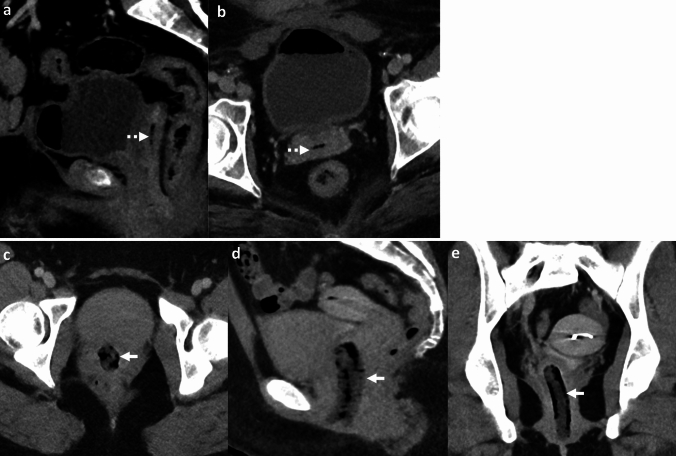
Pitfalls for air in vagina. a, b Intravaginal gas: Patient with hysterectomy and cystitis with recent Foley’s catheterization. CT shows trace air within remnant vaginal canal (dotted white arrow, a, b). c–e Vaginal tampon: A 21-year-old with abdominal pain. CT images show incidental vaginal tampon (solid white arrows, c–e). Mottled lucencies are due to air trapped in the cotton/cellulose material of the tampon
